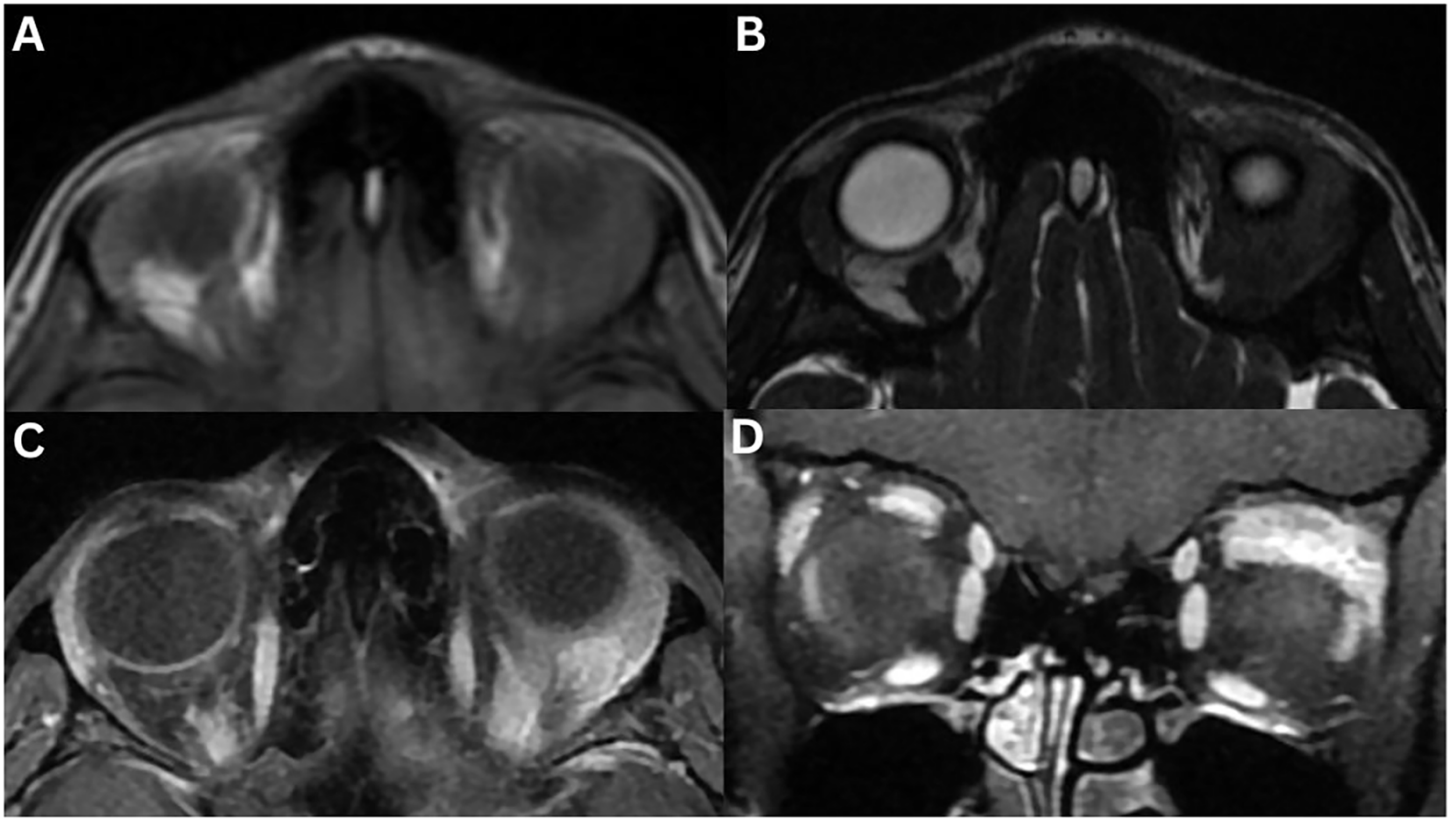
Granulomatosis with polyangiitis involving the left lacrimal gland. MRI T1-weighted axial (A), axial 3D Fiesta-C (B), T1-weighted fat-suppressed contrast-enhanced axial (C) and coronal (D) demonstrate enlargement of the lacrimal gland including the orbital and palpebral lobe. The gland is homogeneous with well-defined margins. It is isointense on T1-weighted imaging and hyperintense on T2-weighted imaging, relative to the extraocular muscles. There is significant enhancement following contrast administration.
